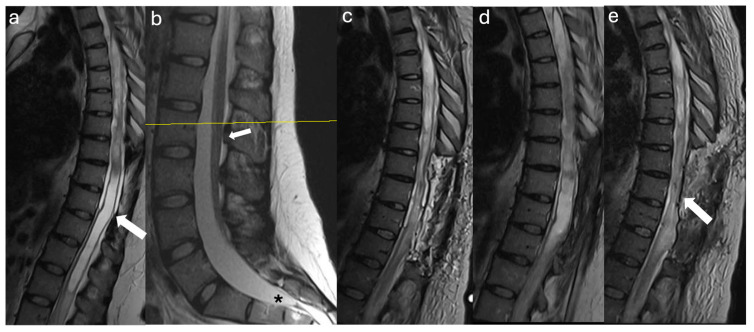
Radiographic Images Case 1: (a) Preoperative sagittal thoracolumbar MRI T2 sequence shows a syrinx extending from the thoracic vertebral level T3 to the conus with visible tethering of the spinal cord to the dura dorsally and ventrally at the mid-syrinx level (arrow). (b) Preoperative sagittal lumbar MRI T2 sequence shows the conus medullaris (arrow) terminating at mid L2 with a visibly thickened filum terminale at the bottom of the thecal sac (asterisk). (c) Postoperative sagittal thoracic MRI T2 sequence before discharge shows laminectomies from T8 to T11 and the expansile duraplasty with marked syrinx reduction at the level and distal to the duraplasty. (d) Three-month postoperative sagittal MRI T2 sequence shows syrinx recurrence despite expansile duraplasty with absence of identifiable focal adhesions. (e) One-month postsyringopleural shunt placement sagittal MRI T2 sequence shows the shunt at the lower syrinx level (T10 level, arrow) with marked reduction in syrinx size.
